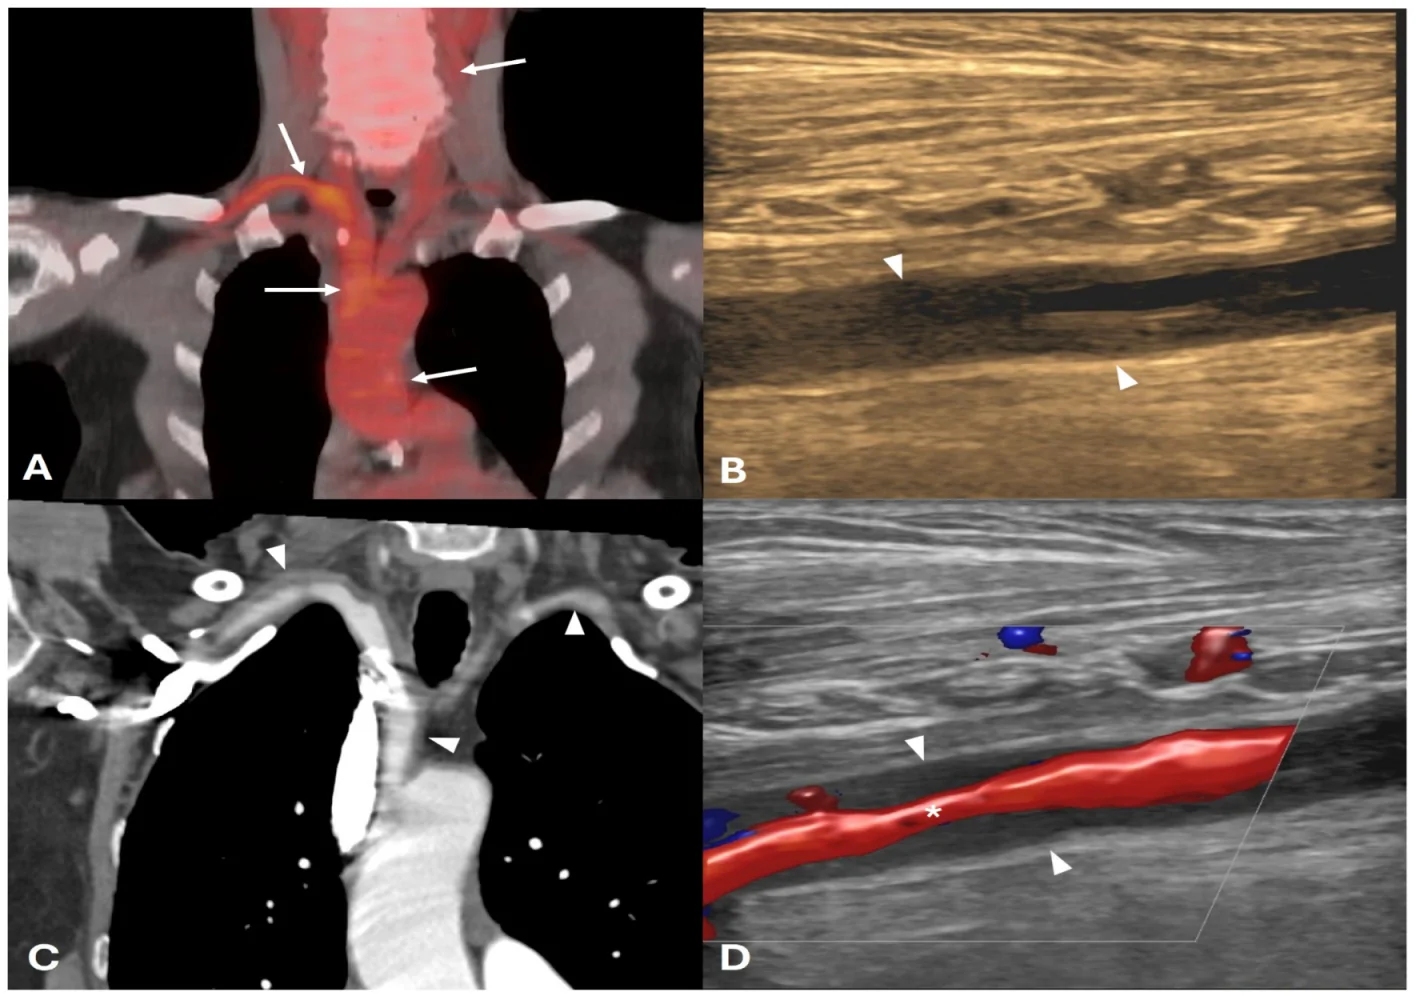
An example of known giant cell arteritis on therapy with elevated inflammatory markers evaluated for disease activity. Coronal fused MPR image of F-18 FDG PET/CT (A,C), gray scale (B) and color Doppler (D) ultrasound images of the right subclavian artery and coronal MPR images of chest CTA. There is abnormal radiotracer uptake involving the ascending aorta, right brachiocephalic, bilateral subclavian and to a lesser extent bilateral common carotid arteries (arrows). In addition, evidence of concentric mural thickening to the brachiocephalic and bilateral subclavian arteries (arrowheads). In the right subclavian artery, there is moderate luminal narrowing (arrowheads), turbulent flow on color doppler (*).
